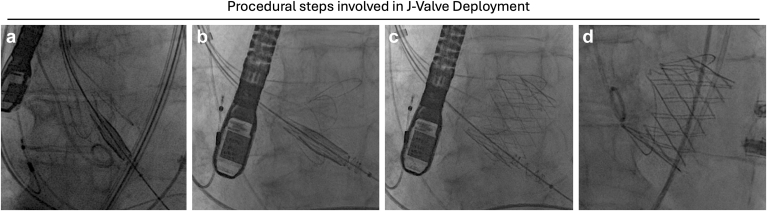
Procedural steps involved in J-Valve deployment. (a) After advancing the J-Valve delivery system into the ascending aorta, the U-shaped nitinol anchor rings are exposed at the level of the sinotubular junction. (b) The anchor rings are advanced to the respective aortic sinuses and confirmed by root angiography and/or transesophageal echocardiography. (c) J-Valve deployed, note the anchor rings are still attached to the control lines. (d) Final fluoroscopy image showing fully deployed J-Valve after release of control lines.
